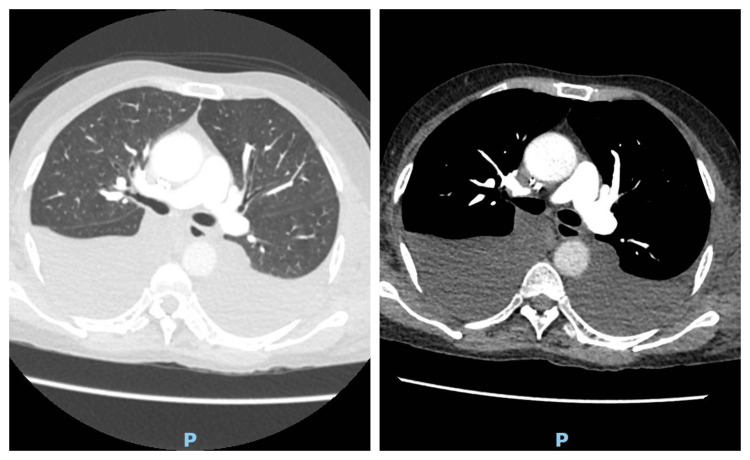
CT chest (lung and mediastinal windows) showing bilateral pleural effusion.
